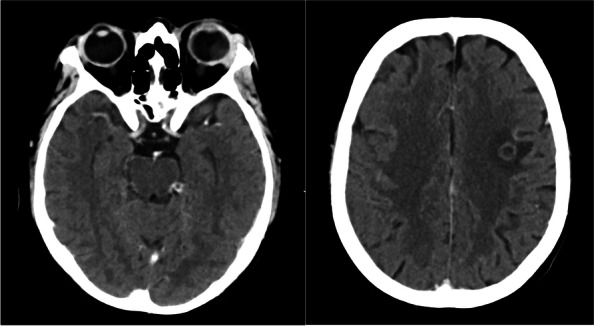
Left image*: Axial contrast-enhanced CT scan of the orbits showing generalized scleral thickness of the left eye with heterogeneous increase in density of the vitreous content. Also seen is soft tissue swelling, orbital proptosis, peri- and post-septal orbital fat stranding plus thickening, and enhancement of the globe wall. Findings that are suggestive of endophthalmitis and subretinal abscess. Enlargement of the lacrimal gland is also noted. *Right image*: Axial section of a contrast-enhanced CT of the brain shows a ring- enhancing lesion in the left frontal lobe measuring 0.9 cm with surrounding vasogenic edema. Findings consistent with intraparenchymal abscess
